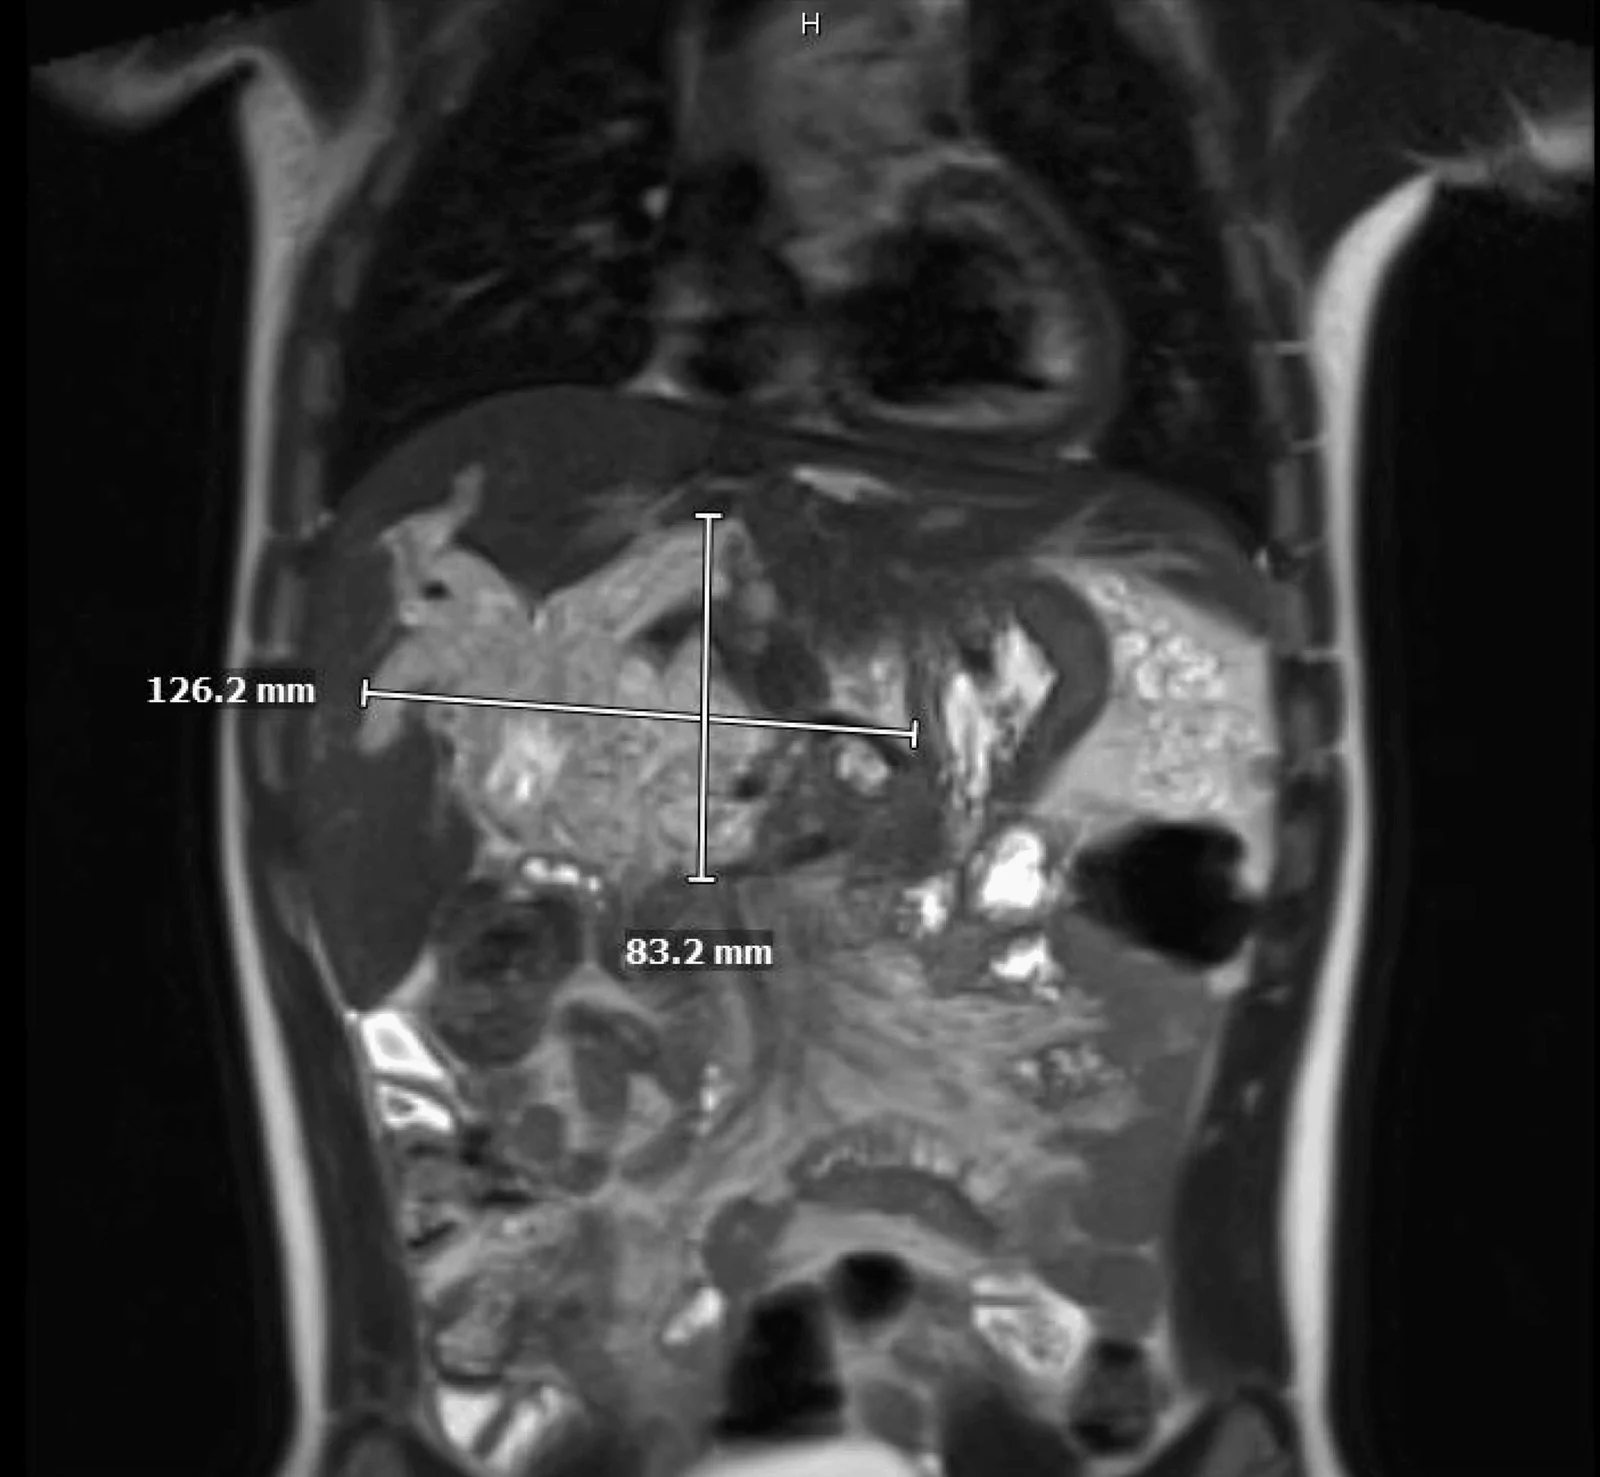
Liver MRI revealing a heterogeneously T2 hyperintense infiltrating mass-like lesion with somewhat tubular components. It is visualized centered in the porta hepatis surrounding the portal vasculature, extending into the liver along the portal vasculature, surrounding the proximal pancreas and celiac axis abutting the IVC, with some mild internal as well as nodular enhancement, suggesting a plexiform neurofibroma. This measures approximately 12.6 × 8.3 cm. IVC: inferior vena cava
